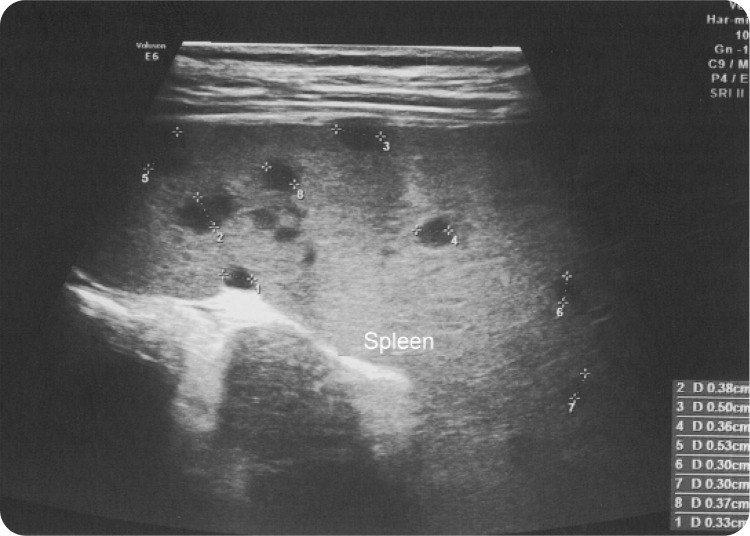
Spleen ultrasound with multiple hypoechoic areas at diagnosis of CSD.
